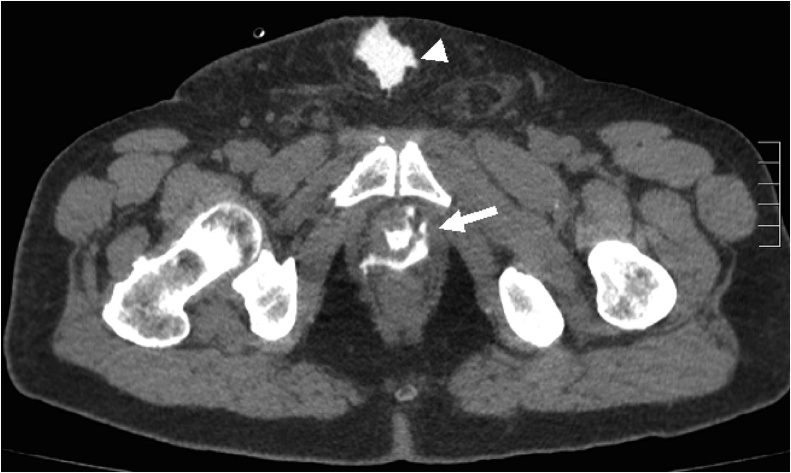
A CT scan showing leakage of contrast at the level of the urethro-vesical anastomosis (arrow), with significant contrast extravasation in the subcutaneous tissue at the midline infraumbilical incision site (arrowhead).
